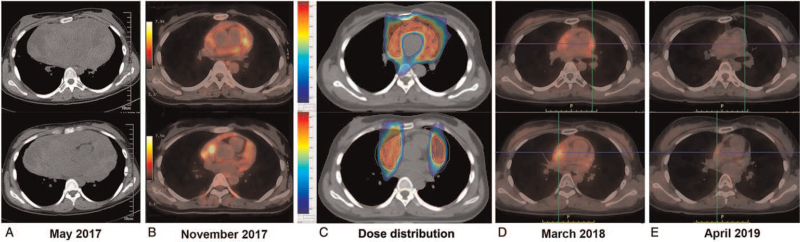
Imaging findings of the tumor during treatment and dose distribution of irradiation target volume. CT scan showed a huge mediastinal mass with invasion of pericardium and great blood vessels before treatment (A). PET/CT revealed the tumor significantly shrank and the SUVmax was 12.6 (B). Colorwash of dose distribution in radiotherapy plan. The red area represents 95% of the PTV receiving a prescription dose of 50 Gy (C). PET/CT indicated small amount of viable tumor tissues existed, SUVmax significantly reduced (SUVmax 1.8–3.9) after radiotherapy (D). PET/CT showed the mediastinal residual mass almost completely diminished and SUVmax was further reduced (SUVmax 1.4) 6 months after the end of apatinib targeted therapy (E).
